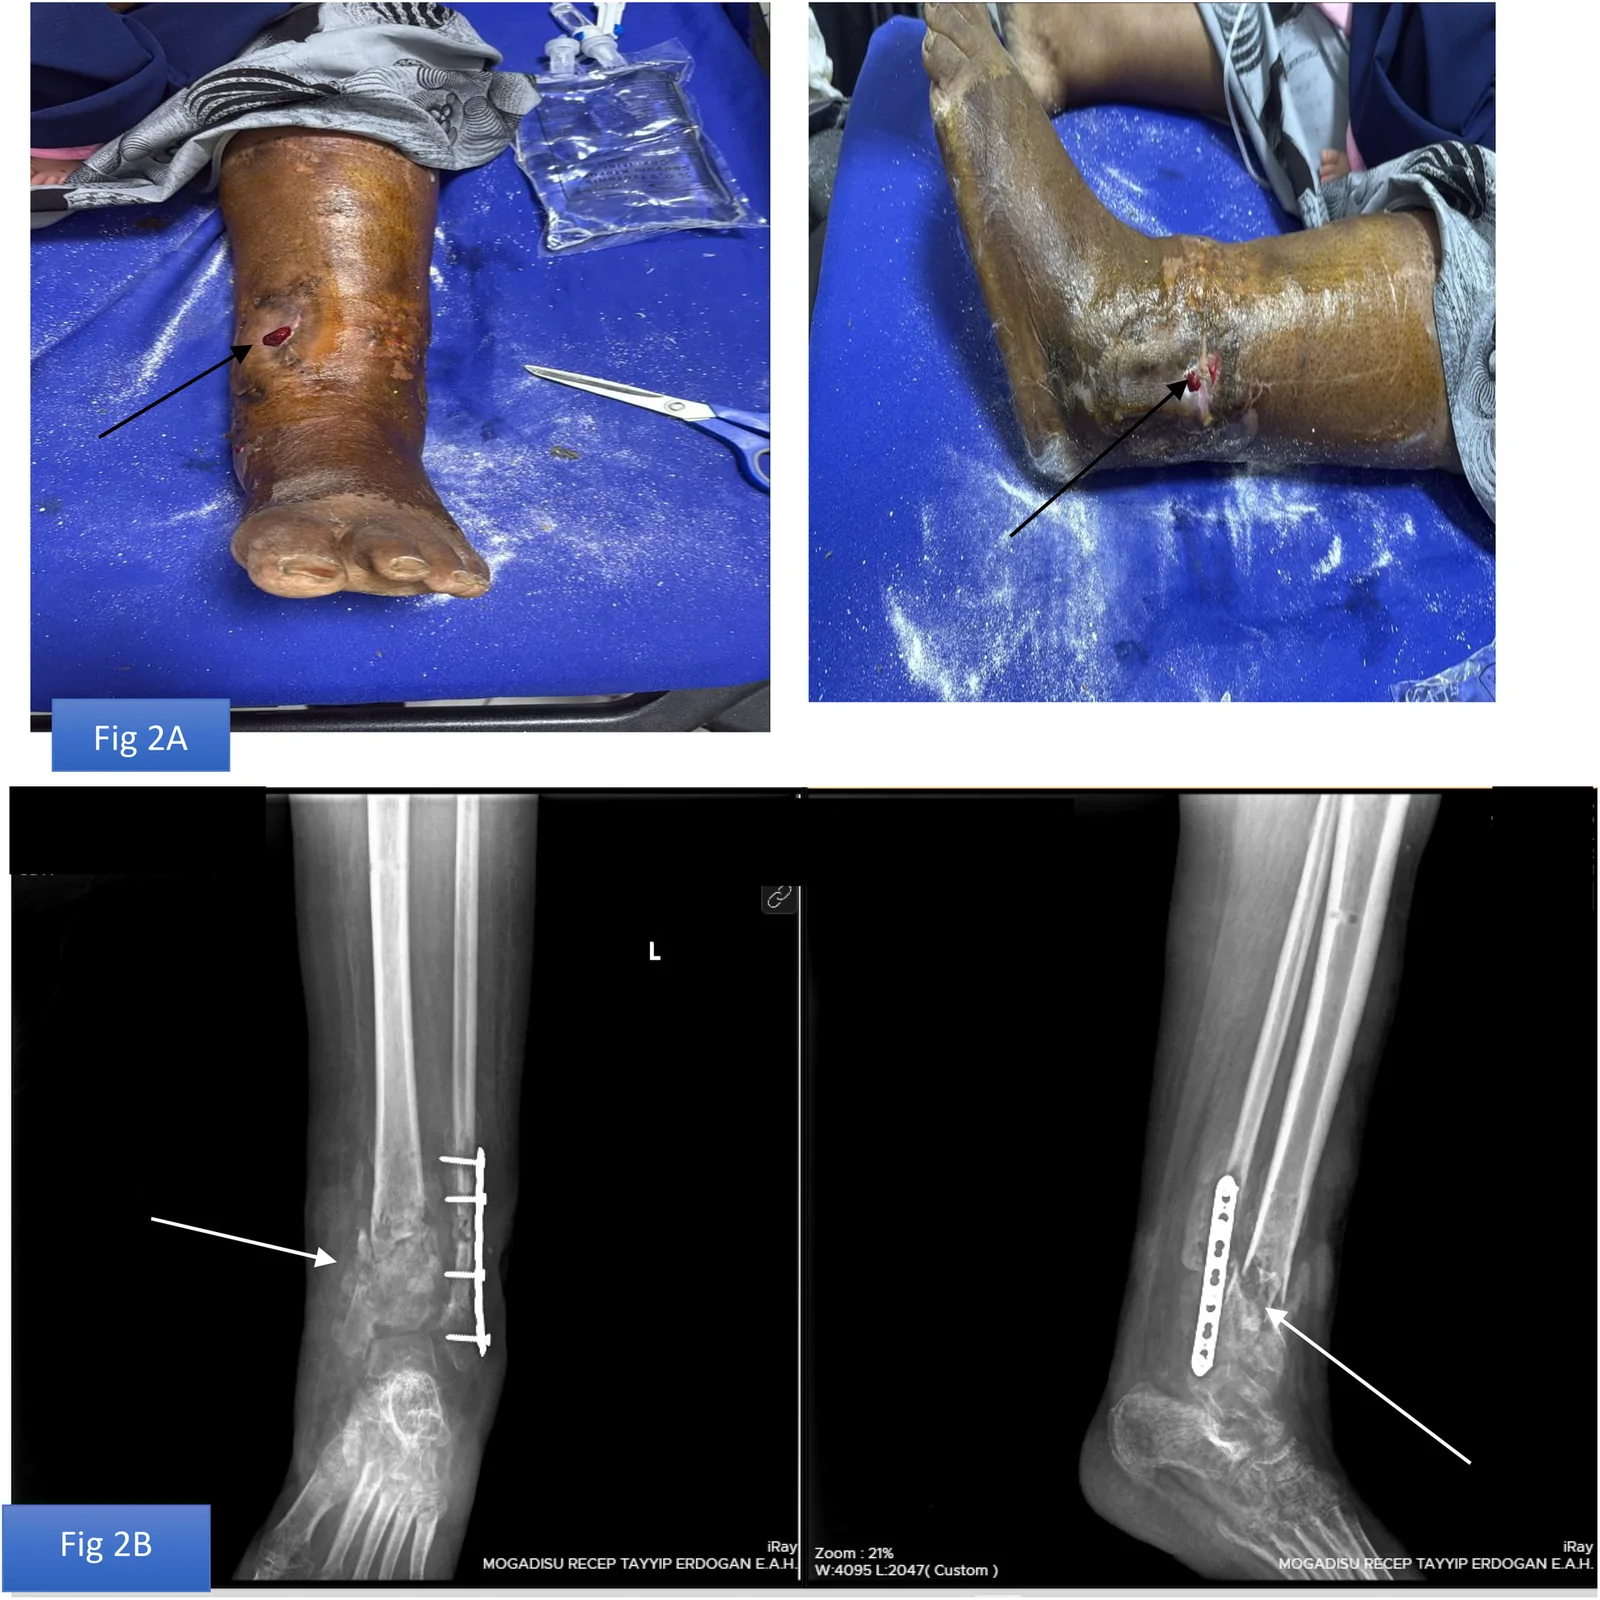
Clinical and radiographic findings at presentation to our institution. (A) Clinical image of the left leg showing a discharging sinus over the distal tibia with purulent discharge, suggestive of infection. The arrow indicates the discharging sinus tract. (B) Radiograph obtained at presentation to our tertiary center showing the previously performed fibular fixation and persistent distal tibial fracture pathology. The arrow indicates the distal tibial fracture region.
